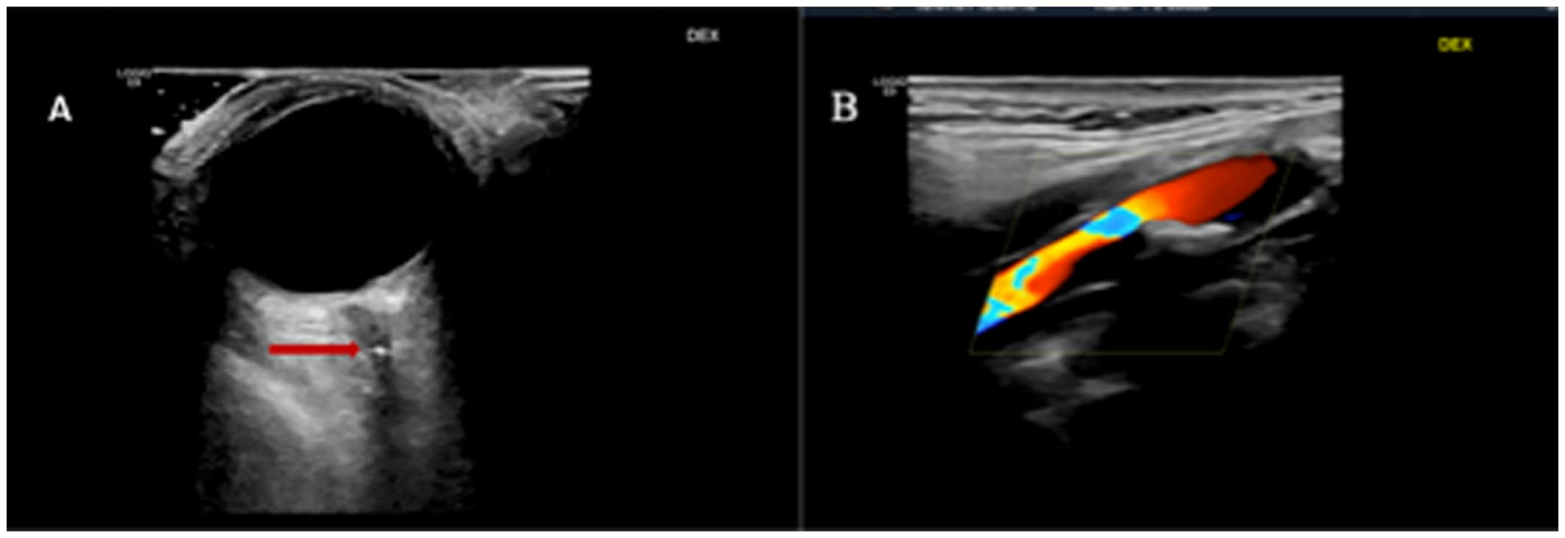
(A) Transorbital ultrasound of the right eye with a “positive spot sign” proximal to the optic nerve head in the central retinal artery (arrow) and (B), right symptomatic carotid stenosis.
